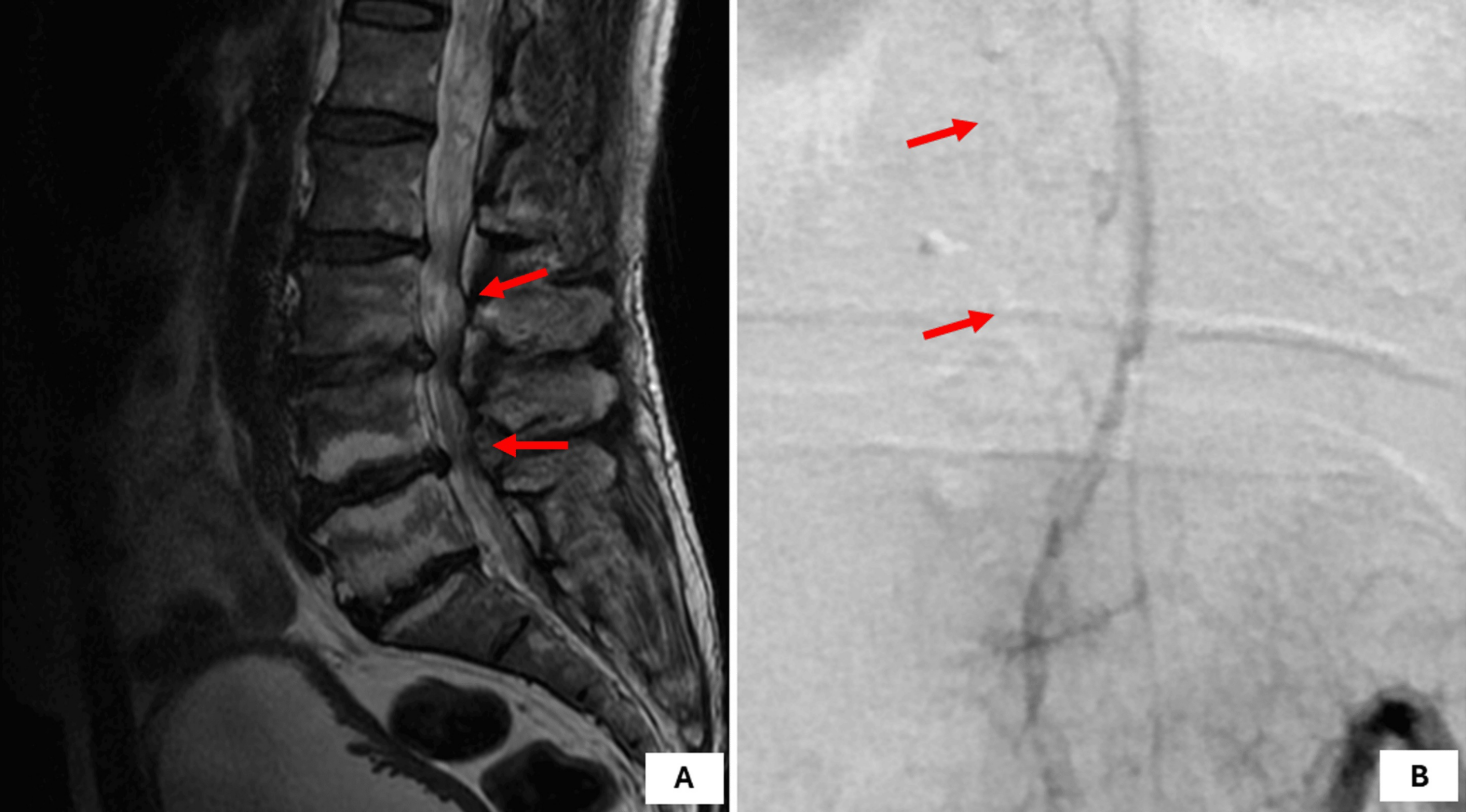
Postoperative (A) MRI and (B) DSA of the patient at the six-month follow-up showing no recruitment DSA: digital subtraction angiography; MRI: magnetic resonance imaging
